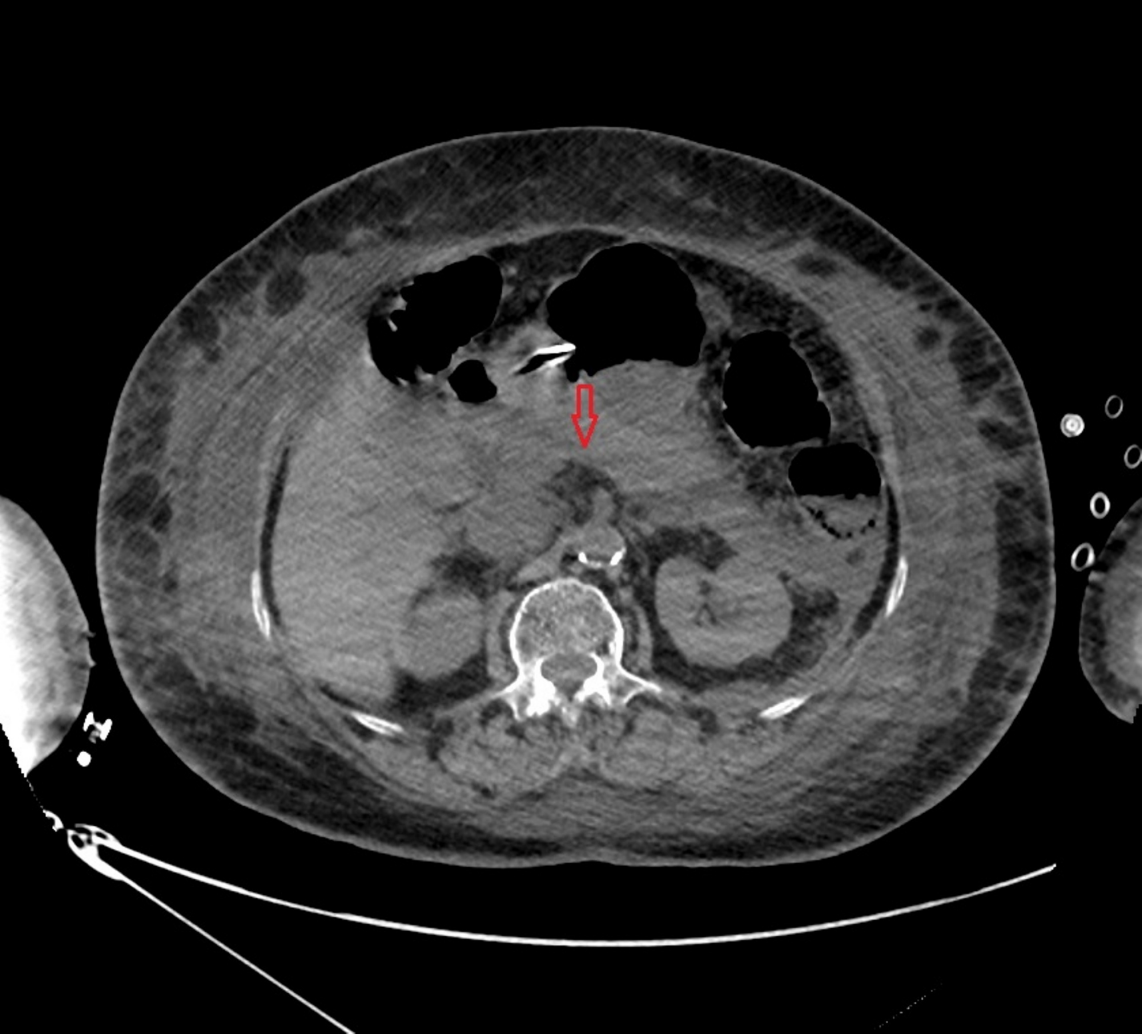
Computerized tomography (CT) scan of the abdomen revealing an enlarged pancreas with inflammatory fluid surrounding it, consistent with acute pancreatitis
